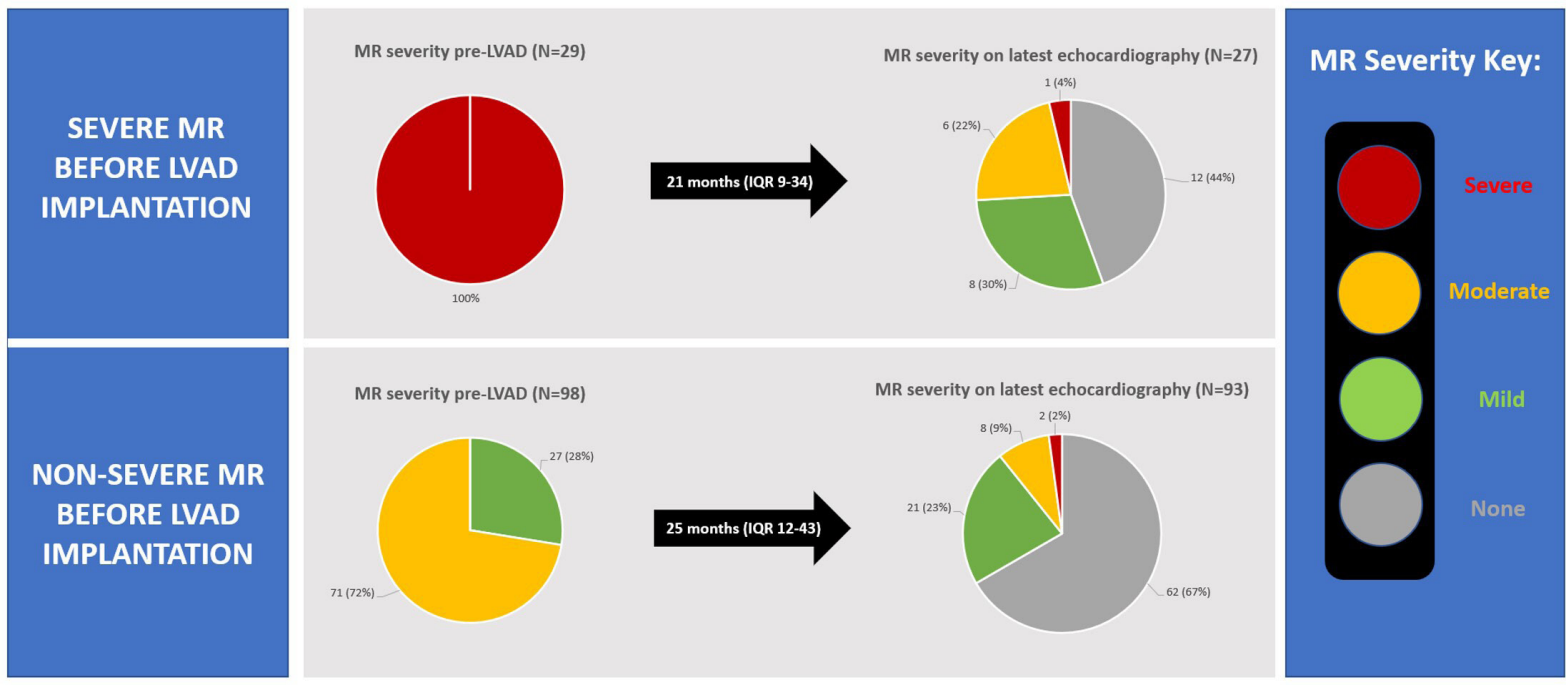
Pie charts demonstrating change in MR severity among patients with severe MR pre-LVAD and non-severe MR pre-LVAD (left). The pie charts on the right demonstrate the relative severities of MR on latest echocardiography. LVAD, left ventricular assist device; MR, mitral regurgitation.
